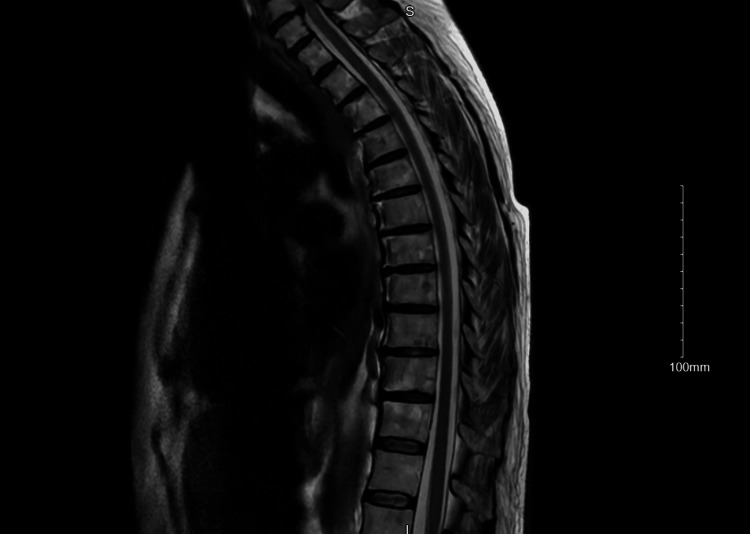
MRI spine complete without and with contrast No discrete lesion in the spinal cord. Multilevel degenerative changes.
